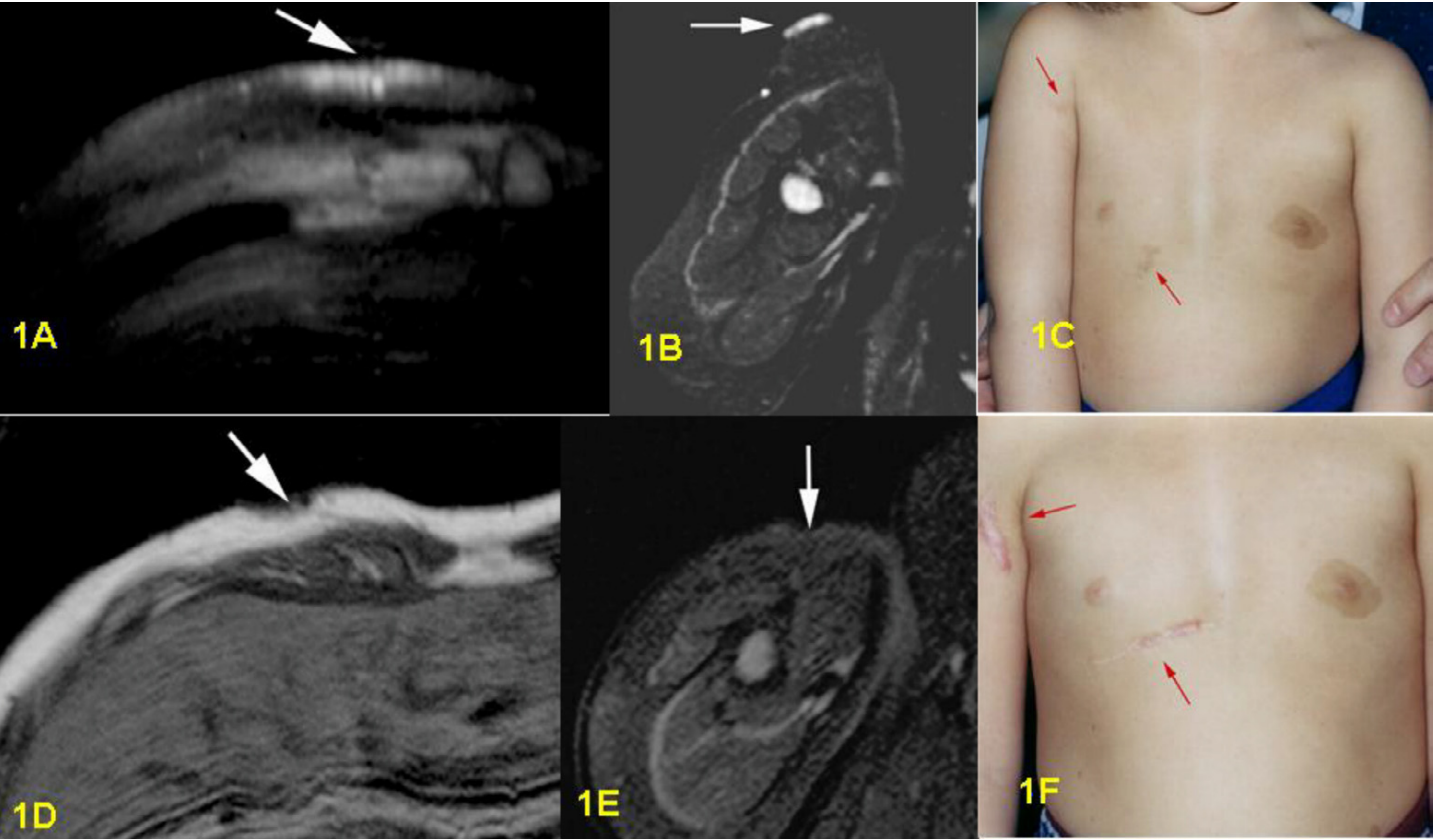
1A: Pre-surgical T2-weighted STIR-sequence, axial section: in right ventral chest wall bright superficial thickening of cutis and subcutis without involvement of muscles. 1B: Pre-surgical T2-weighted STIR-sequence, axial section: very bright, flat superficial cutaneous PNF in proximal part of right upper arm. 1C: Pre-surgical clinical frontal view of both PNF on right upper arm and thorax wall. 1D: Post-surgical T1-weighted sequence, axial section: small defect in cutis and subcutis, no PNF visible anymore. 1E: Post-surgical T2-weighted STIR-sequence, axial section: complete removal of tumor with hypointensive induration. 1F: Post-surgical clinical frontal view of both scars on right upper arm and thorax wall.
